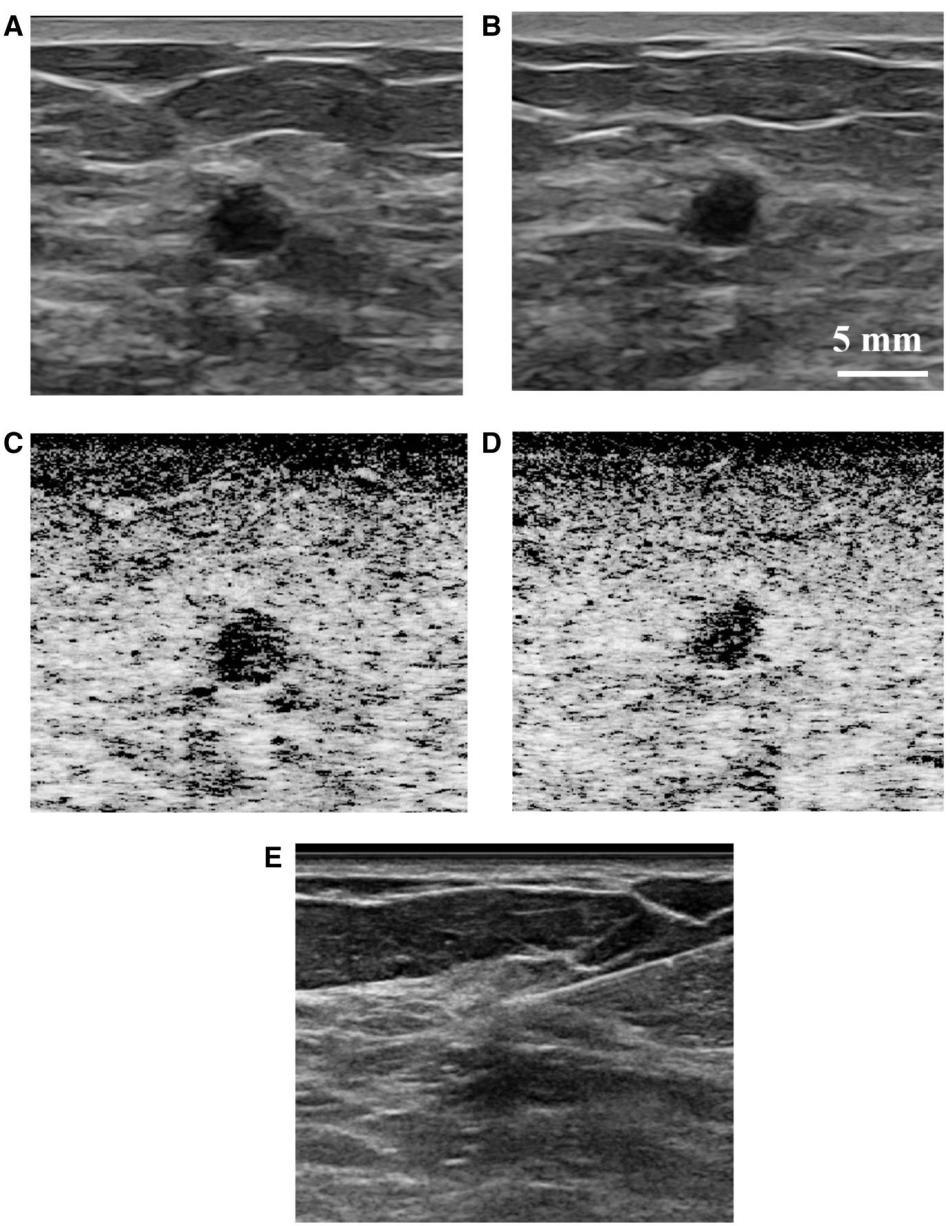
Images from a 66-year-old woman called back from screening mammogram for a left breast mass. Pairs of B-mode ultrasound static images show a 5 mm solitary circumscribed mass vs complicated cyst seen in the (A) antiradial and (B) radial projections at 1 cm depth from the ultrasound probe. (C) Antiradial and (D) radial SLSC images of the same finding show darker (or less coherent) lesion content relative to the surrounding tissue. One out of 6 board-certified radiologists characterized the finding as fluid based on B-mode ultrasound images presented in the reader study, and 5 out of 6 board-certified radiologists characterized the finding as fluid based on the SLSC images. The 1 radiologist who characterized the finding as solid with B-mode images and uncertain with SLSC images, drew regions of interest that resulted in a gCNR of 0.91 for the SLSC image, which categorizes the finding as fluid. Ultrasound-guided aspiration was performed with the (E) post-aspiration image demonstrating complete aspiration of the finding yielding benign-appearing fluid, consistent with a benign complicated cyst.
